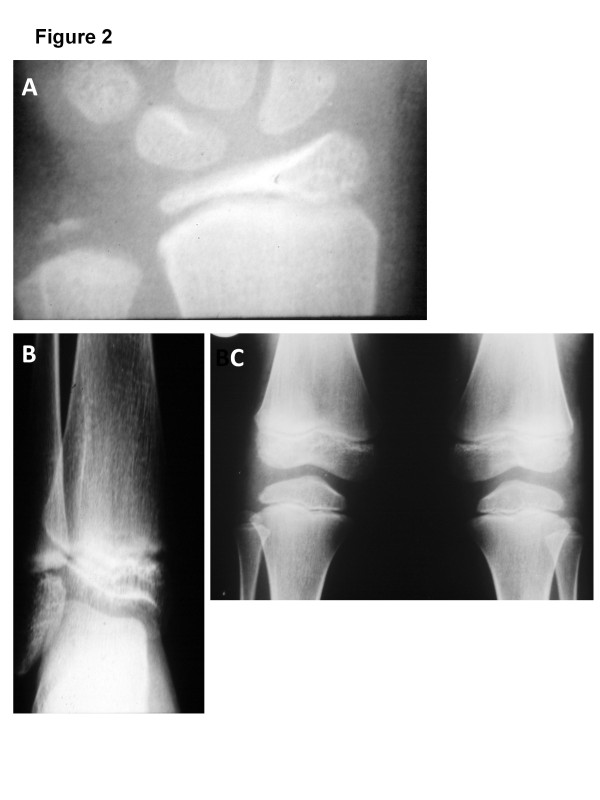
X-ray images of some affected members. A) AP film of the wrist in case IV-2 at the age of 10 years showing flattening of the radial epiphyses and underdeveloped ulnar epiphyses. B) AP film of the ankle in IV-2 at ten years of age, showing flattening of the tibial epiphyses. C) AP film of the knee in case IV-4 at six years of age showing flattening of the tibial and femoral epiphyses with some irregularities of the end plates of femoral epiphyses.
